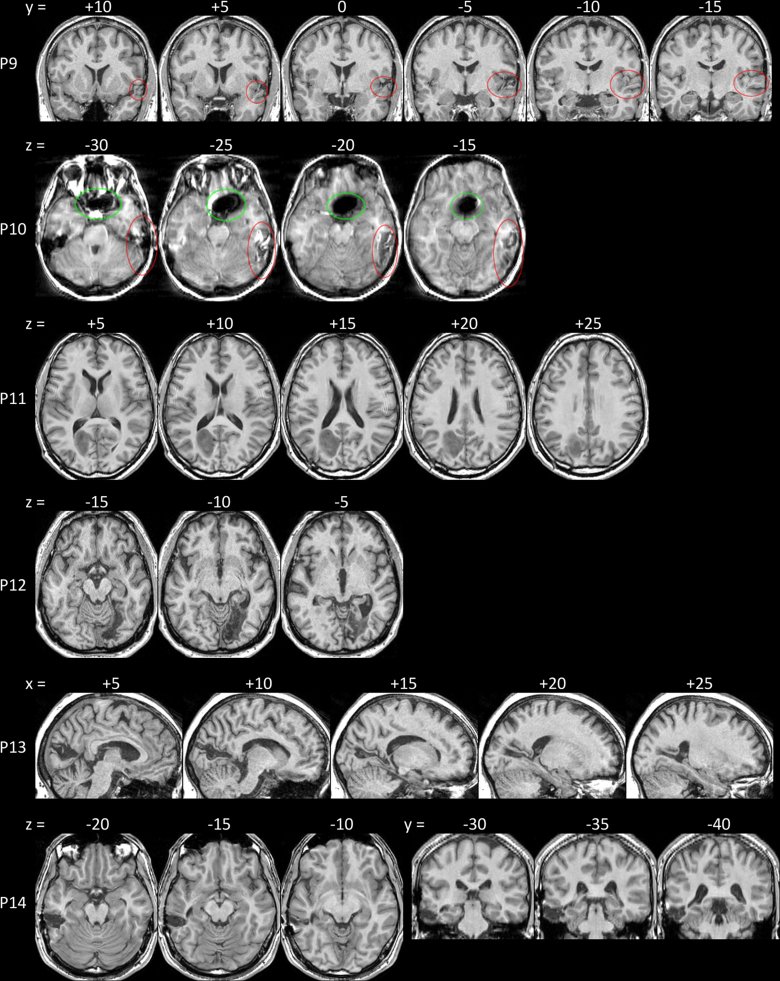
The vATL-intact patients' lesions shown on T1-weighted structural scans normalised to MNI space. For P9 & P10, the lesions are circled in red to aid location. Green circle: image artefact caused by aneurism clip. See Table 1 for lesion descriptions. MNI *y* and *z* coordinates are reported above each section. Images are shown in neurological convention with patient's left on image left.
